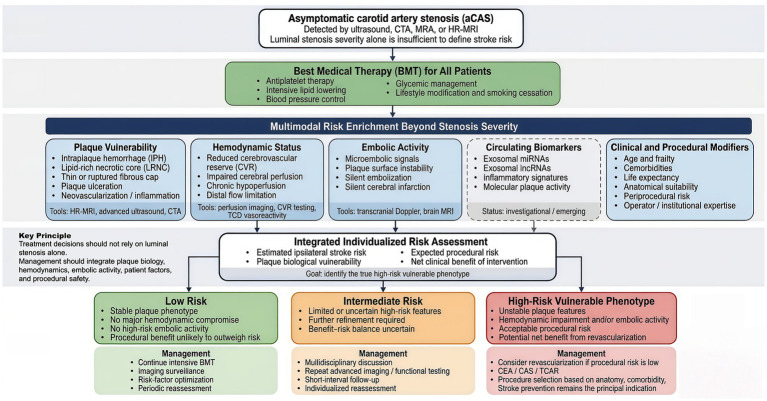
Individualized precision risk stratification framework for asymptomatic carotid artery stenosis. Clinical application of multimodal biomarkers should be guided by risk stratification. Standard carotid ultrasound and routine blood tests are indicated for all patients with aCAS. Advanced imaging (HR-MRI, perfusion imaging, CVR testing, TCD) and liquid biopsy (exosomal miRNAs/lncRNAs) should be reserved for selected subgroups, including those with: Ultrasound features suggestive of plaque vulnerability (hypoechoic plaque, ulceration, rapid progression); Prior contralateral stroke or TIA; Uncertain risk–benefit balance for revascularization; Younger age or life expectancy >5 years. PET imaging may be considered in research settings to assess plaque metabolic activity.
